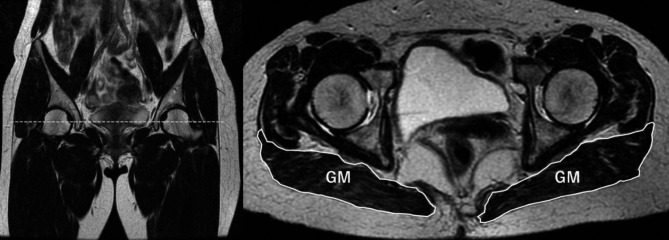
Cross-sectional areas (CSA) of the gluteus maximus (GM) CSA of the GM were obtained bilaterally at the center of the femoral head on a T2-weighted hip axial MR image
